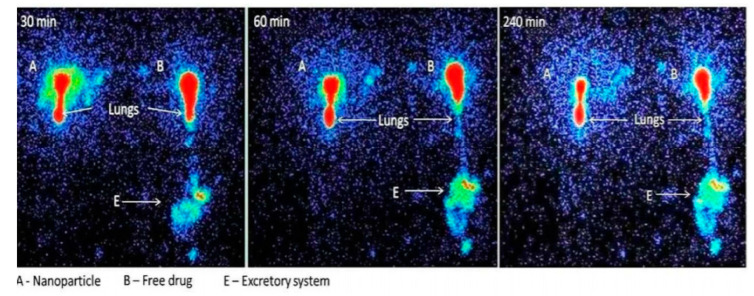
Gamma scintigraphy showing that 4 h post administration of free voriconazole accumulated in the excretory system whereas VNP remained in the lungs [64].
